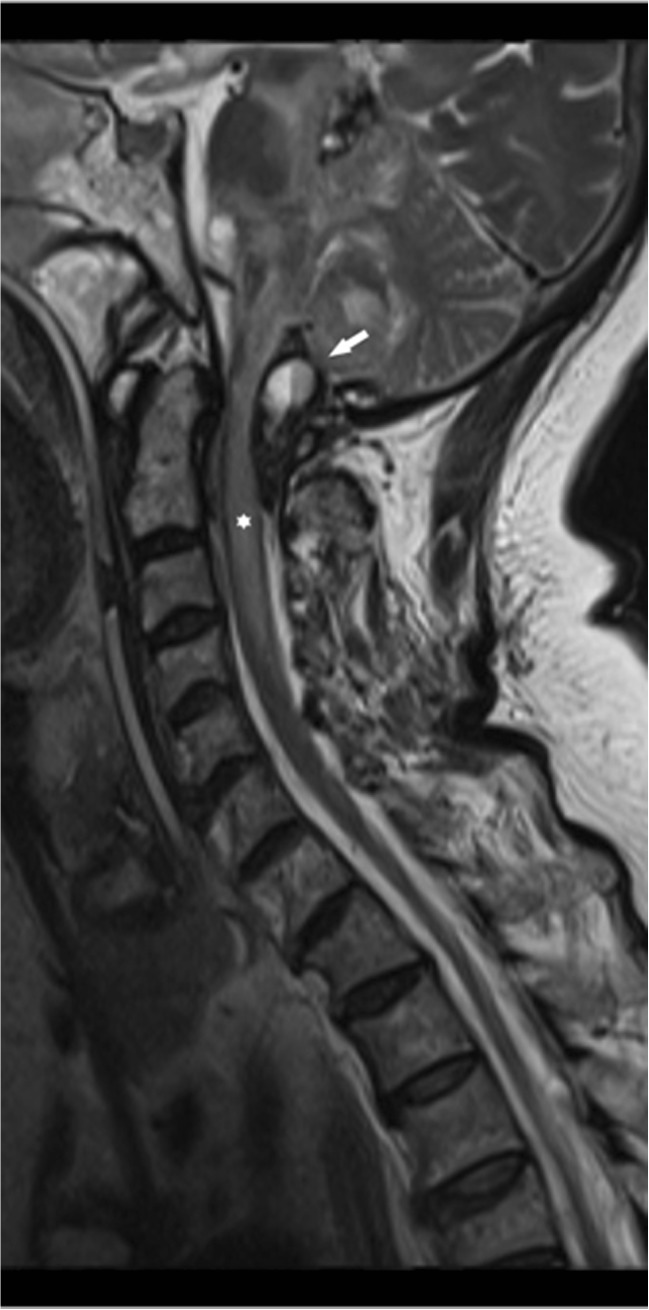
Sagittal T2-weighted MR image of the cervical spine. An epidural mass with fluid level, consistent with an acute hemorrhage, is compressing the lower brain stem and upper cervical spinal cord at the level of the foramen magnum (*white arrow*). Additionally, hemorrhage is present at the level of the supracerebellar cistern. Note an increased signal intensity in the entire brain stem, upper cervical spinal cord (to the level of C4) (*white asterisk*), and cerebellum
